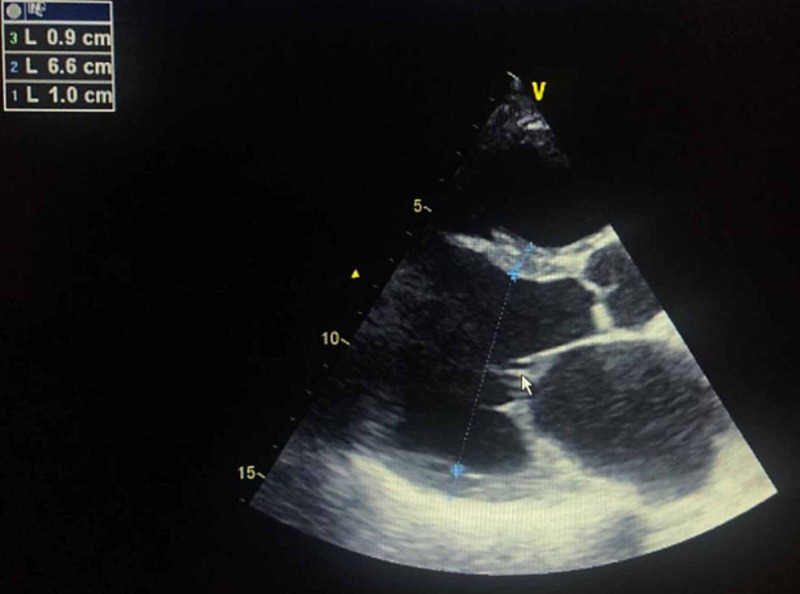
Transthoracic echocardiography showing left ventricular dilatation
